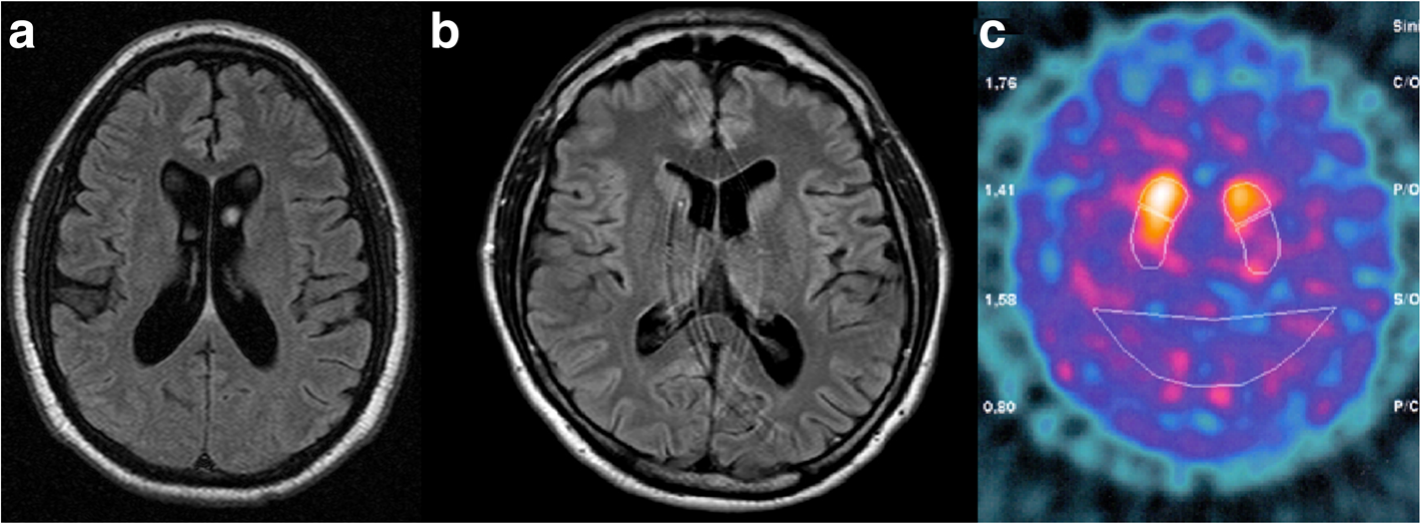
a FLAIR axial brain MRI exhibiting subtle cortical atrophy; (b) FLAIR axial brain MRI exhibiting mild cortical atrophy (c) Dopamine transporter scan (DaTScan) exhibiting bilateral decrease in tracer uptake, greater in the left putamen and, to a lesser extent, caudate nuclei
